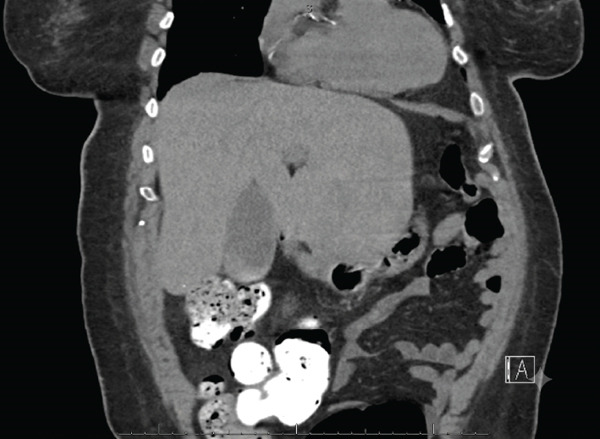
Abdominal CTA coronal view confirmed the presence of a focal hypodense lesion in the distal ileal loop near the ileocecal valve measuring up to 1.9 cm.
